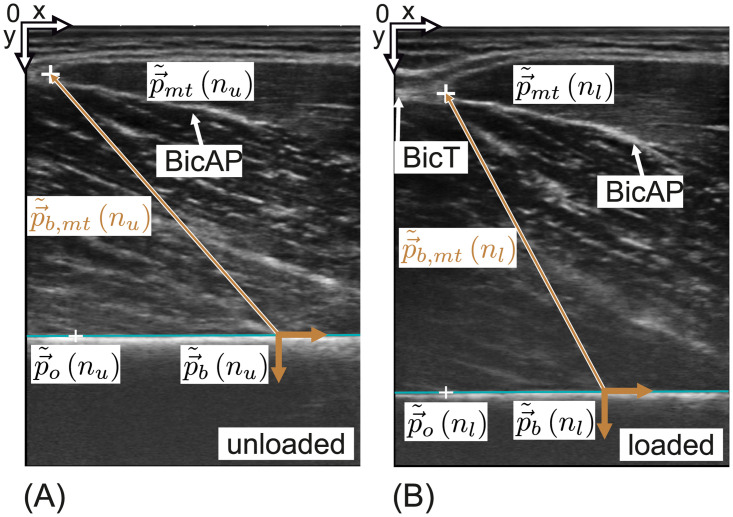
Ultrasonic images of the myotendinous junction (white cross) in the parasagittal plane for the (A) unloaded and (B) loaded case (cmp. Fig 4A, orientation I) are shown. (A) shows the unloaded state *n*_*u*_ (*F*_*w*_ = 0) and (B) the loaded state *n*_*l*_. Reference points ${\tilde{\vec{p}}}_{b}$ on the *humerus* and ${\tilde{\vec{p}}}_{mt}$ on the myotendinous junction are marked in the frame of each state. The reference points were used to calculate the position of the myotendinous junction ${\tilde{\vec{p}}}_{b,mt}$ relative to the bone local coordinate system (brown). To calculate the bone surface orientation within the frame, a second marker ${\tilde{\vec{p}}}_{o}$ was placed arbitrarily on the bone surface. That is ${\tilde{\vec{p}}}_{o}\left(n_{u}\right)$ for the unloaded state and ${\tilde{\vec{p}}}_{o}\left(n_{l}\right)$ for the loaded state. The bone line was set such that it included both bone points ${\tilde{\vec{p}}}_{o}$ and ${\tilde{\vec{p}}}_{b}$. The bone line is drawn in turquoise for each frame.
